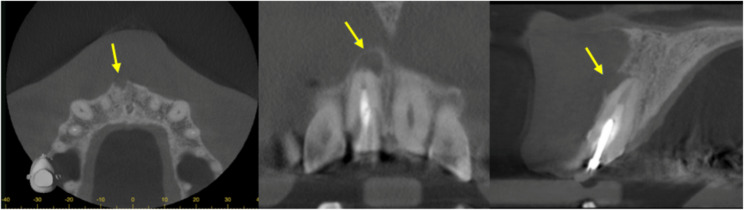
View of periapical defect in 3 axes. The yellow arrow indicates the area of bone destruction associated with the periapical lesion resulting from failed root canal treatment. This site is also where the parasite was detected
